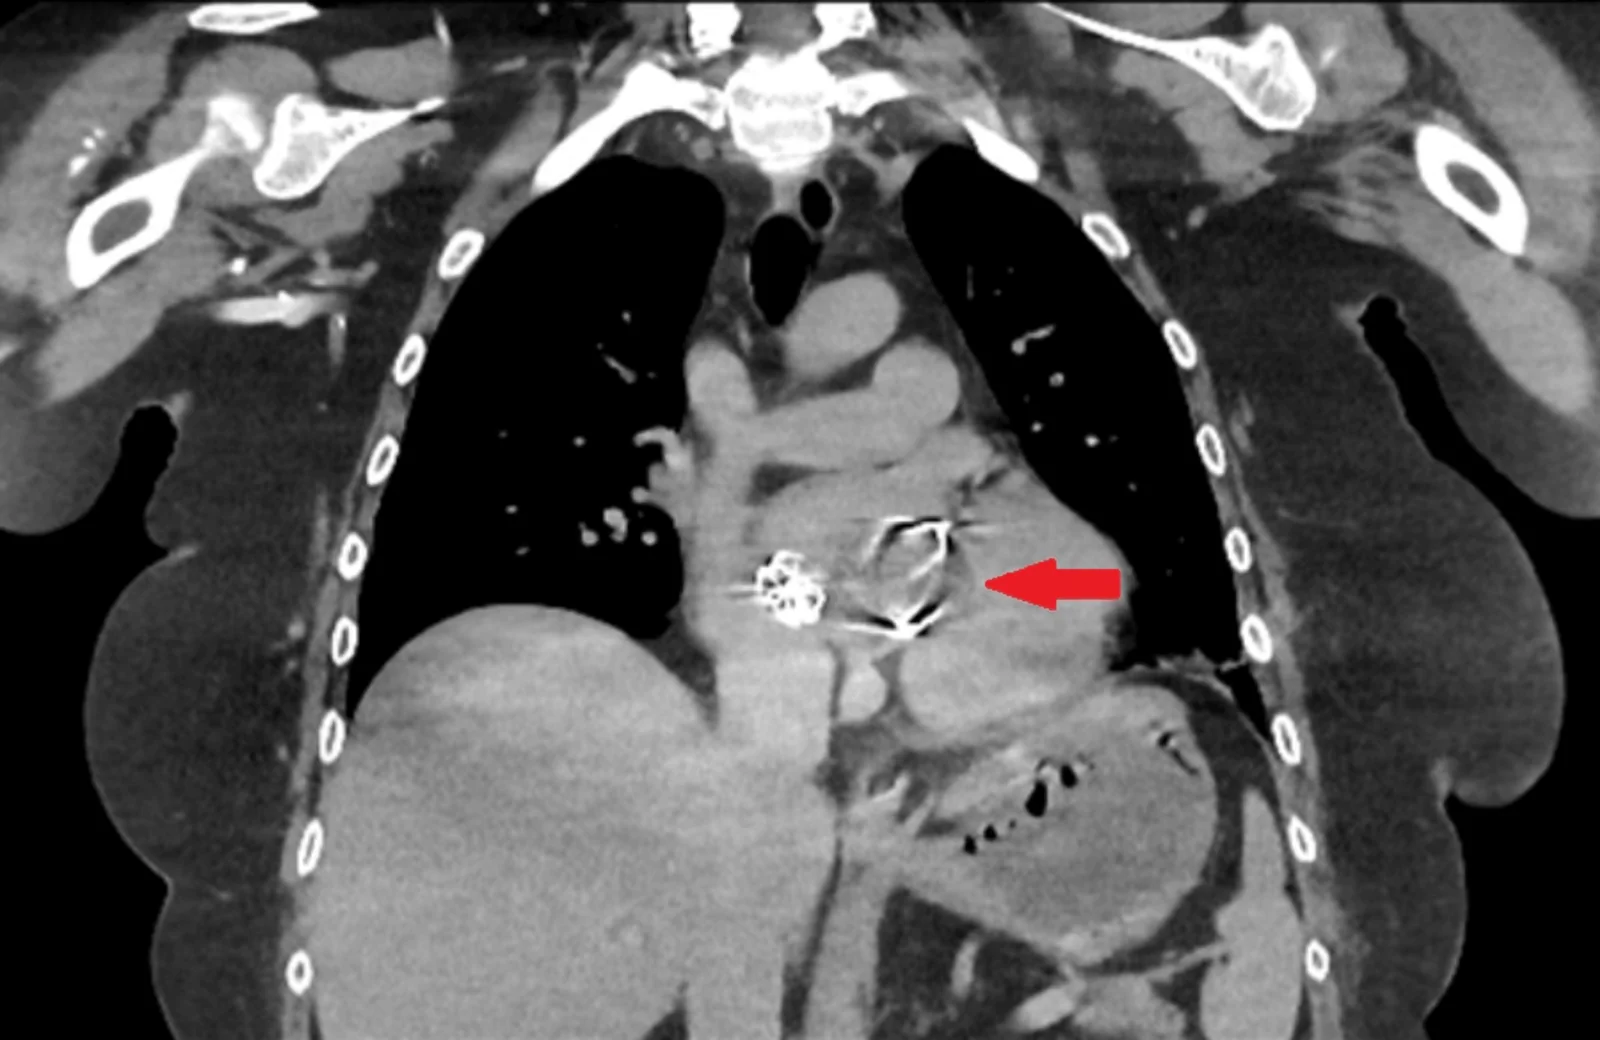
Coronal contrast-enhanced computed tomography (CT) image of the thorax demonstrating metallic artifact from the mitral valve repair (red arrow).
